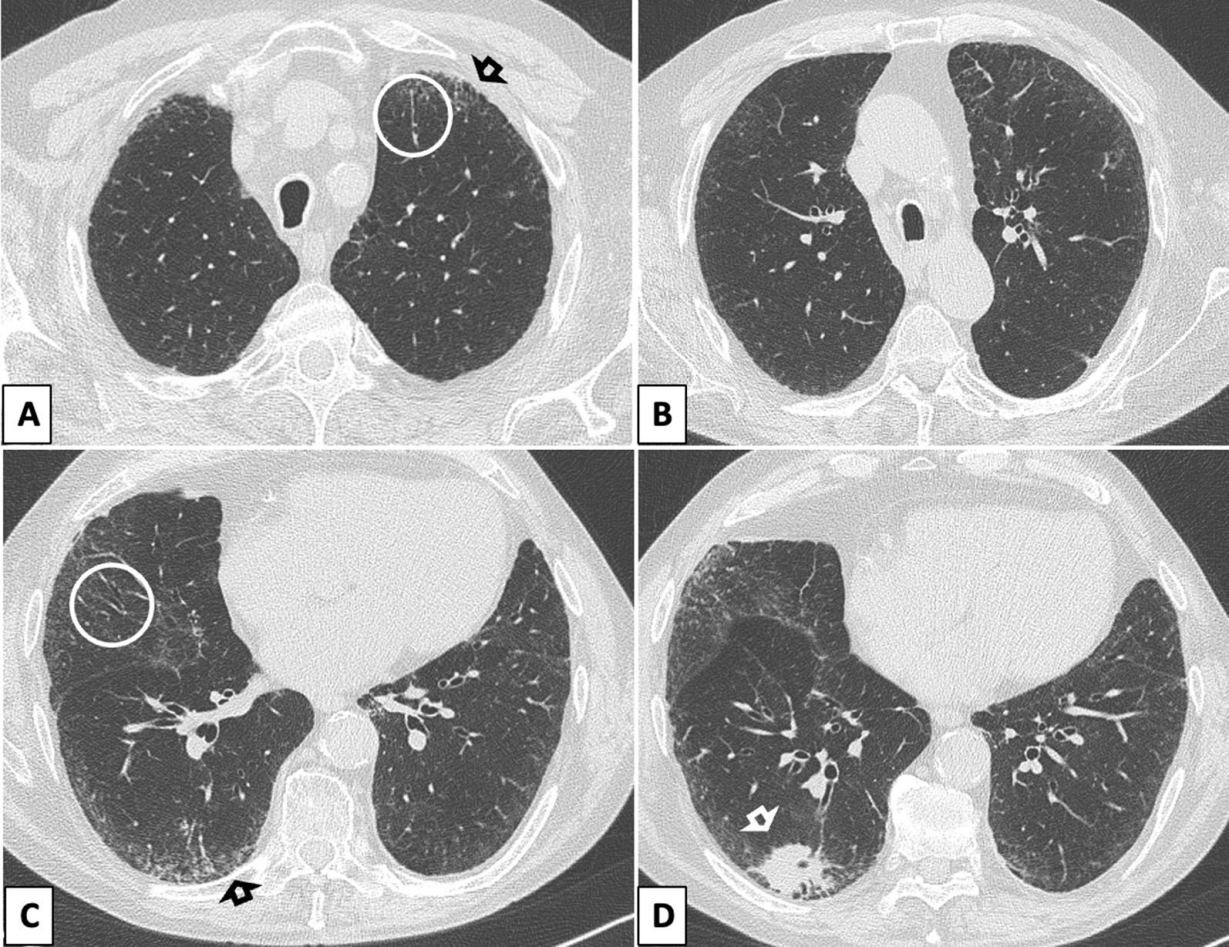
a–d Axial CT images (a–d) without contrast (slice thickness of 1 mm) of an 81-year-old gentleman show traction bronchiectases (*white circles*) and subtle cystic airspaces (*black arrows*), compatible with subpleural fibrotic ILA. (d) The CT scan, performed following the depiction of a nodular consolidation in the right lower zone at chest radiography, also showed a mass in the right lower lobe (white arrow) highly suspicious for primary lung cancer
